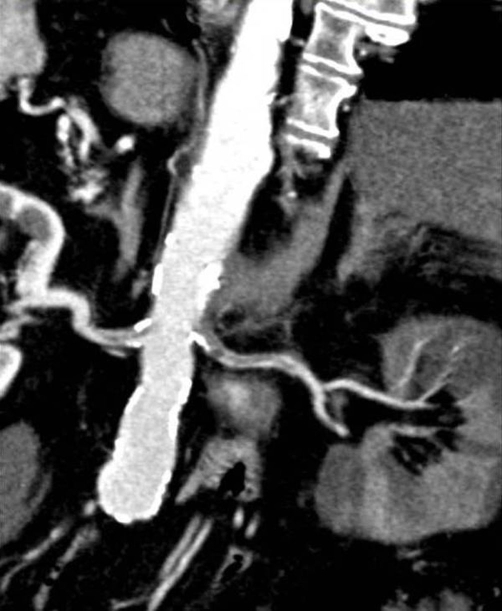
CT image of subocclusive thrombus of right renal artery (right arrow) showing renal hypoperfusion (diminished cortical contrast enhancement). Left arrow shows a floating trhrombus in left renal artery.
